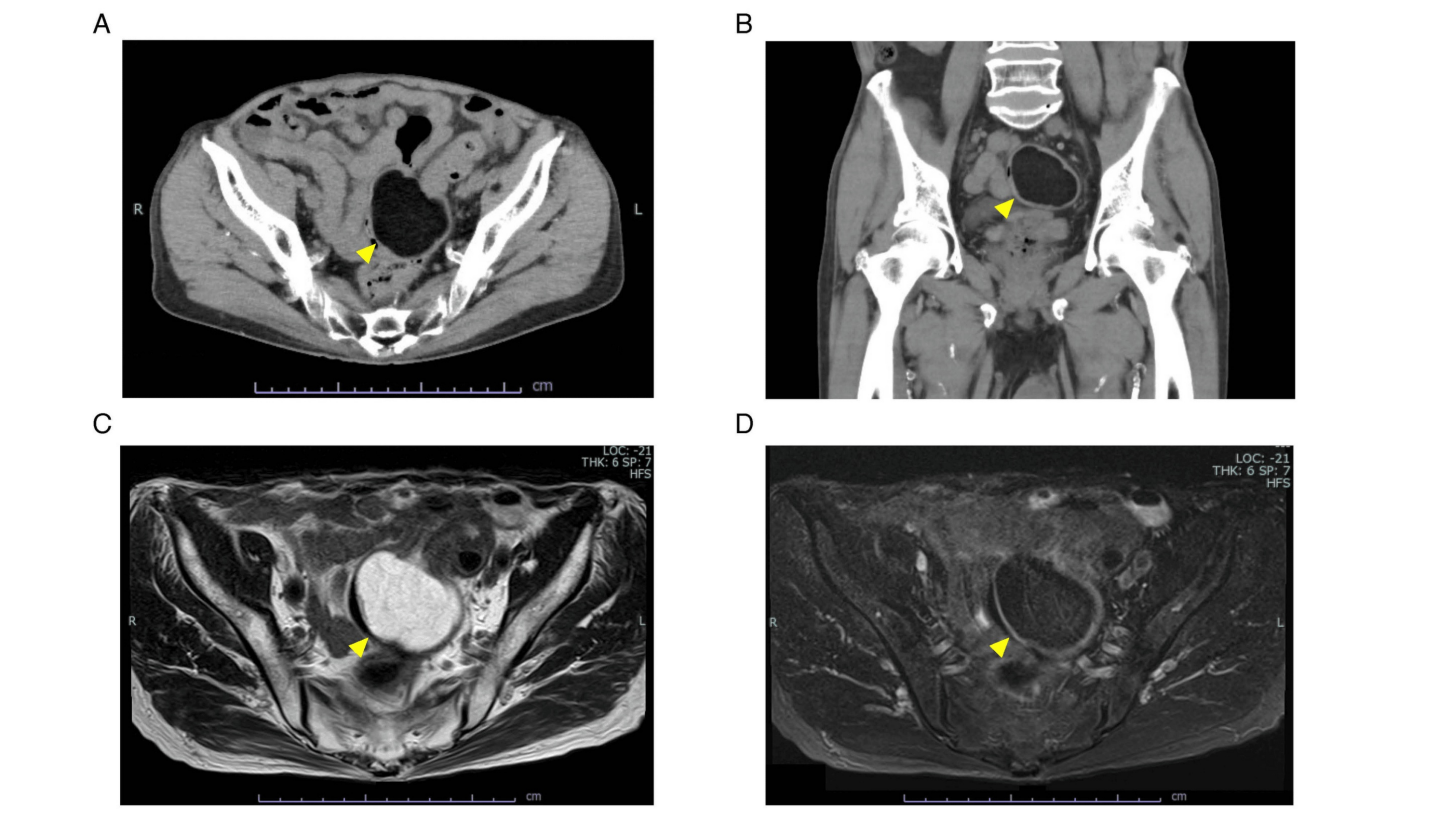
Preoperative imaging findings of the sigmoid colonic lipoma (A, B) CT images reveal a well-demarcated, low-density lesion in the sigmoid colon, consistent with adipose tissue. (C, D) MRI further confirms the lipomatous nature of the lesion with high signal intensity on T1-weighted images and fat suppression. Arrowheads indicate the tumor in all panels (A–D).
